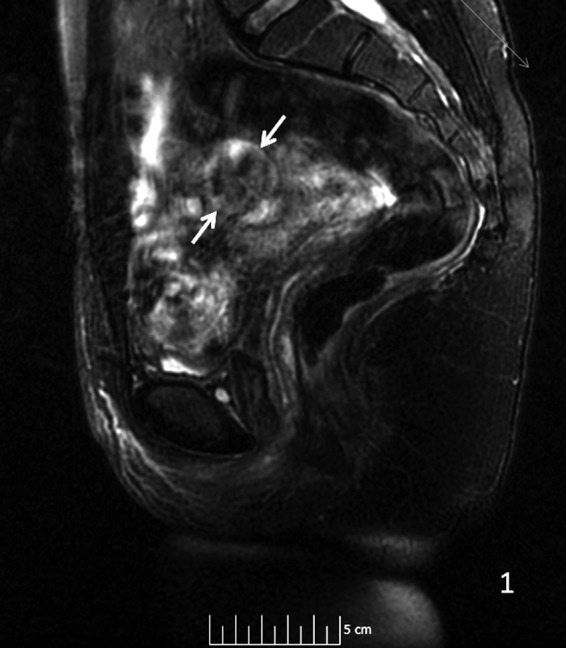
Sagittal T2 MRI shows arrows pointing to an in homogeneous pattern in the mass. MRI, magnetic resonance imaging.
